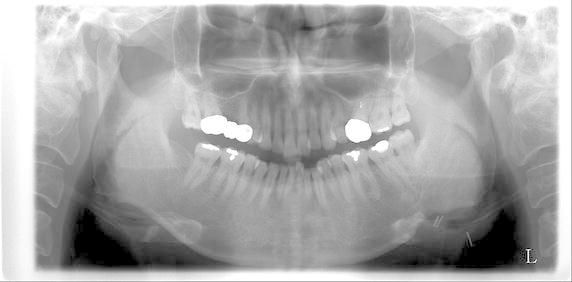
Postoperative panoramic radiography. The bone healing was evident in the left mandibular notch area at 5 months postoperatively
